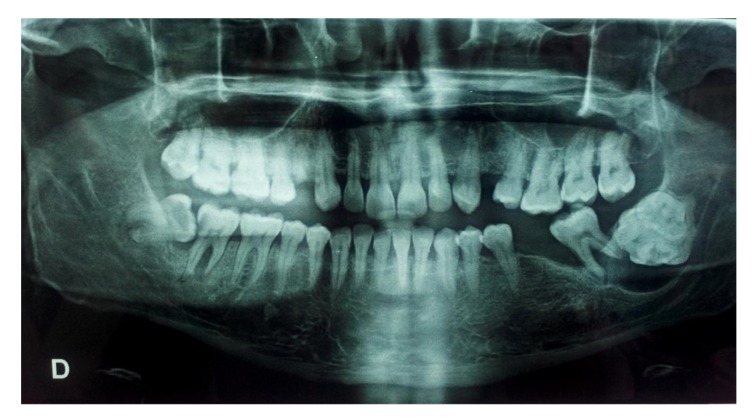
Panoramic radiograph showing the lesion as well defined radiopacity in the left side of the mandible in the angle region.
